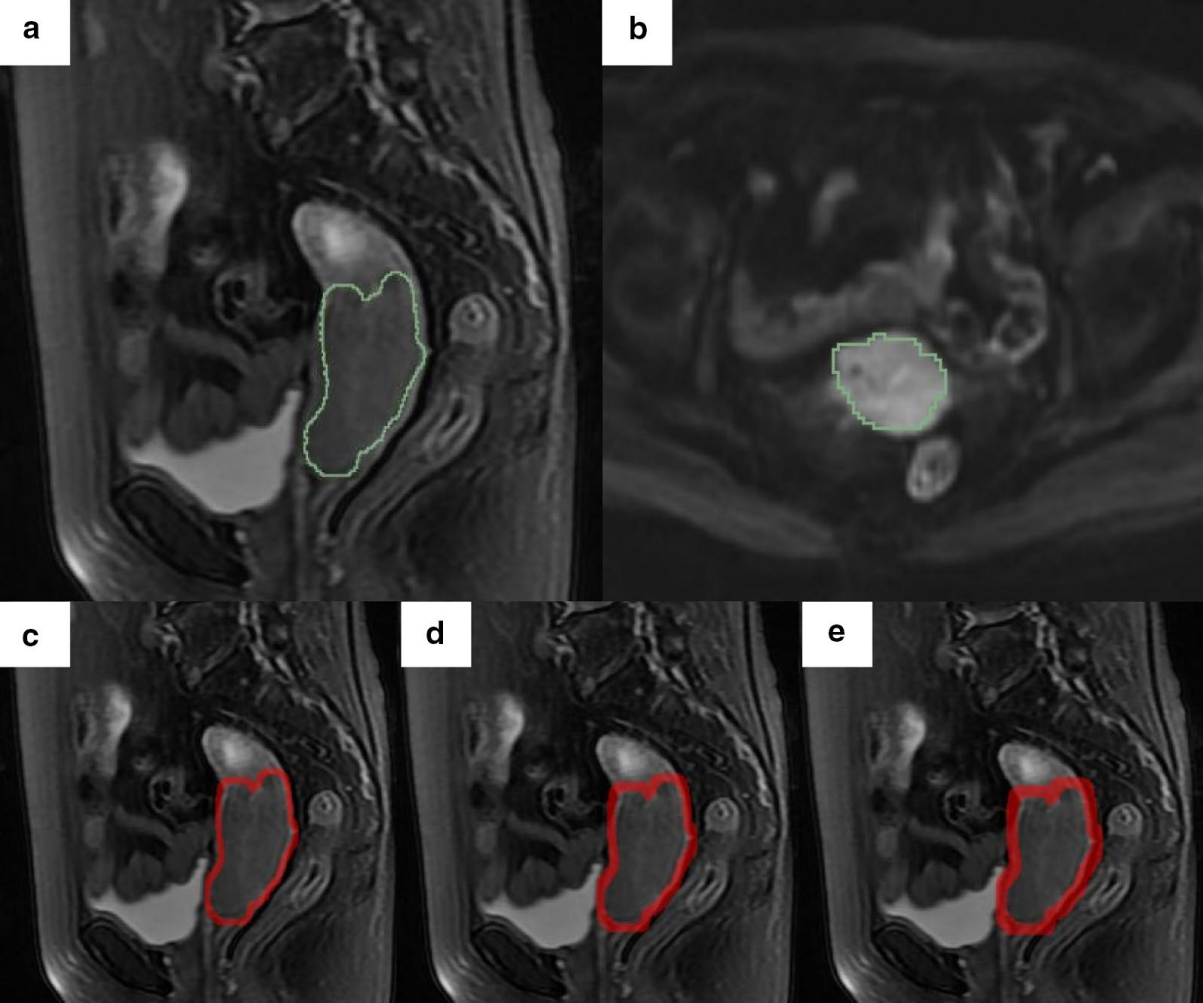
The intra- and peritumoral ROIs in this study. a is on sagittal T2WI; b is on DWI. The green line represents the boundary of the tumor; c-e are the example (3, 5, 7 mm expansion dimension) of the dilated MRI with various radial dilation distances outside the original masks in the MRI. The red rings indicate the peritumoral regions
